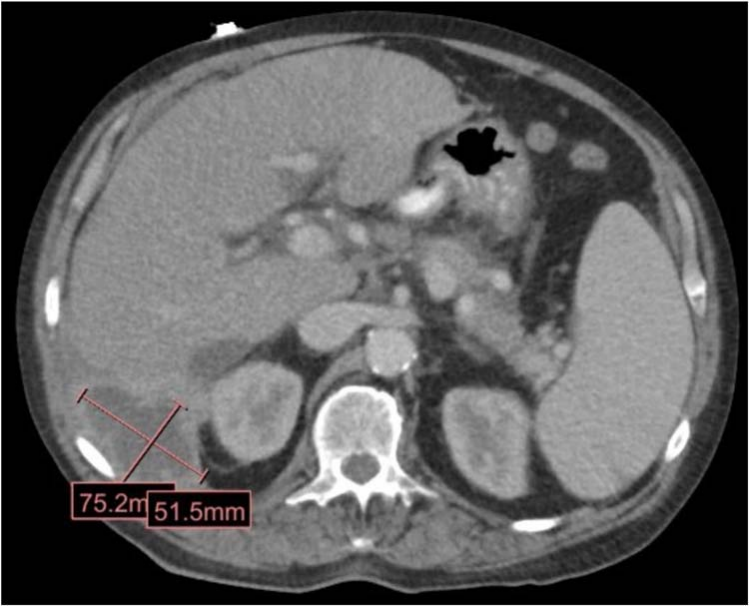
CT in the transverse plane demonstrating a perihepatic gallstone-containing collection of fluid measuring up to 7.5 × 5.1 cm.
